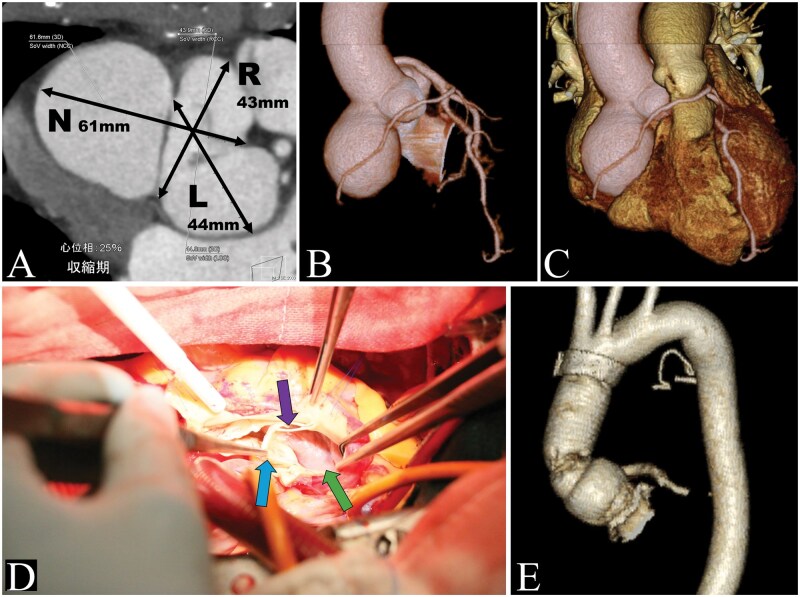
Preoperative Imaging, Intraoperative Findings, and Postoperative Computed Tomography. (A) Preoperative contrast-enhanced computed tomography at the level of the sinus of Valsalva demonstrates a 61-mm aneurysm of the non-coronary sinus. (B) Preoperative 3-dimensional reconstructed computed tomography image. (C) Anatomical findings of a single coronary artery in which the right coronary artery originates from the left main trunk and courses anterior to the pulmonary artery. (D) View of the aortic root during inspection. The non-coronary sinus side is being observed. Blue arrow: non-coronary cusp. Green arrow: The inner wall of the sinus of Valsalva, which is thinned and appears pale pink. Purple arrow: fenestration of the valve cusp. (E) Three-dimensional reconstruction image from postoperative contrast-enhanced computed tomography. Abbreviations: L, left coronary sinus; N, non-coronary sinus; R, right coronary sinus
